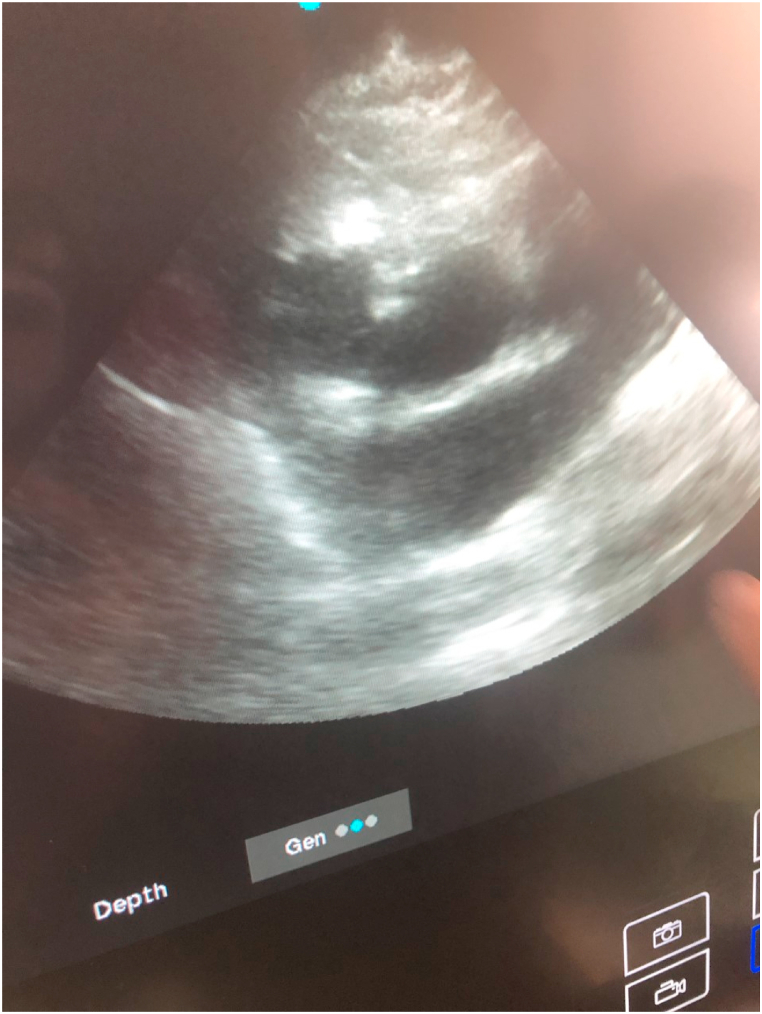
Left atrial mass and right ventricular dysfunction seen on bedside cardiac ultrasound.
